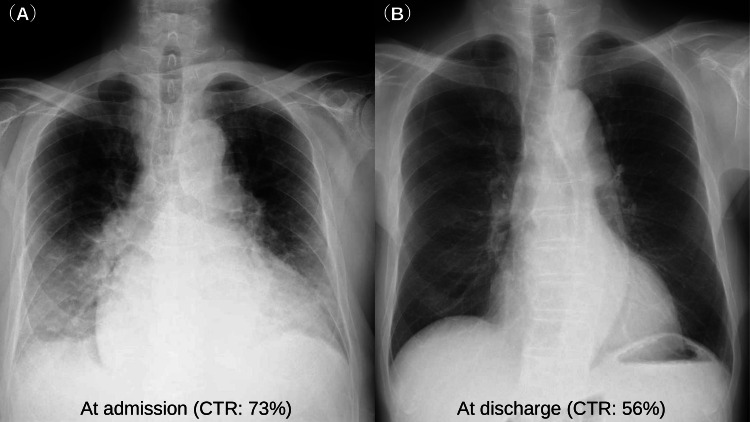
Chest X-ray at the time of admission and discharge. Chest X-ray showed cardiomegaly (CTR: 73%) and pulmonary congestion at the time of admission (A). The CTR decreased to 56% and pulmonary congestion disappeared at the time of discharge (B).
